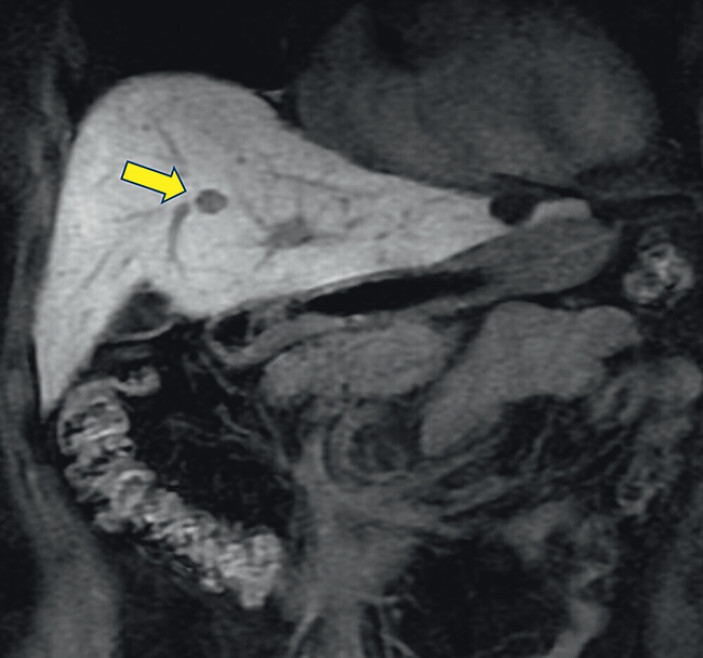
Abdominal contrast-enhanced magnetic resonance imaging revealed a 16-mm lesion in segment 4 of the liver (arrow).
